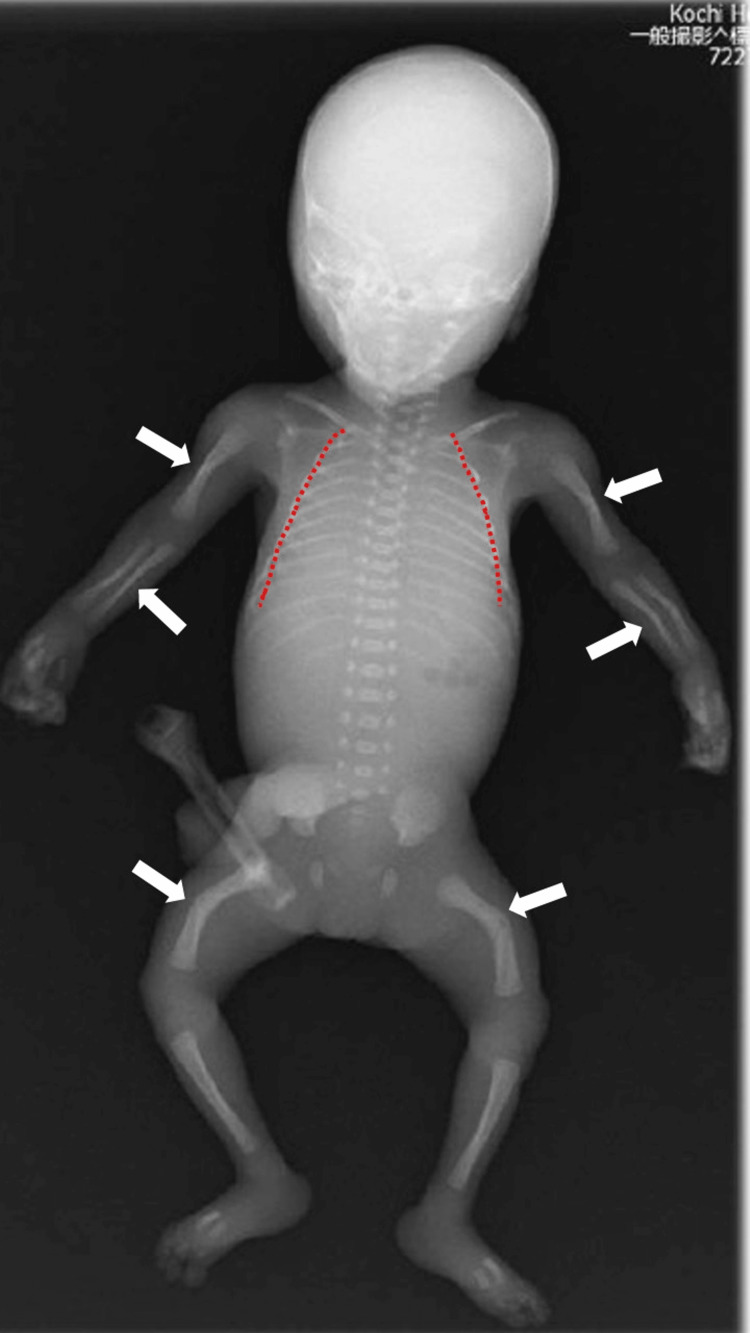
X-ray of the neonate's entire body Humerus, forearm, and femur kyphosis were noted (white arrow), with a small thorax (red dotted line).
